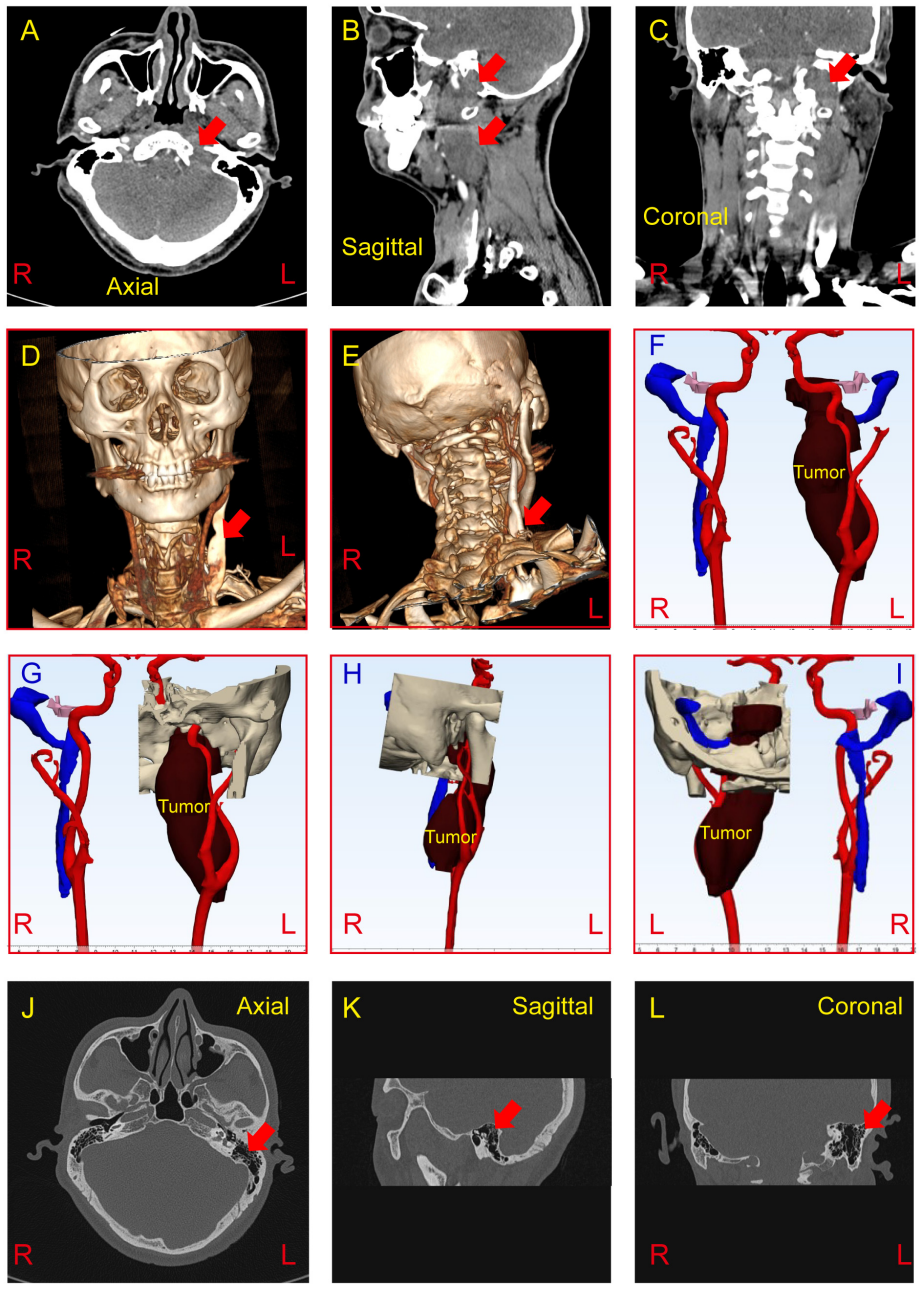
(A-C) CT imaging reveals an abnormal density mass in the left cerebellopontine angle, jugular foramen, and retrostyloid space, demonstrating mild to moderate heterogeneous enhancement (Red arrow). The tumor measures approximately 37*20*87mm. (D-I) Three-dimensional tumor model was reconstructed based on preoperative CT scan to show the spatial relationship of the tumor, arteries, and veins. (J-L) CT findings demonstrate no abnormalities in the bilateral external ear, middle ear, and inner ear (Red arrow show the left mastoid). R, Right; L, Light.
